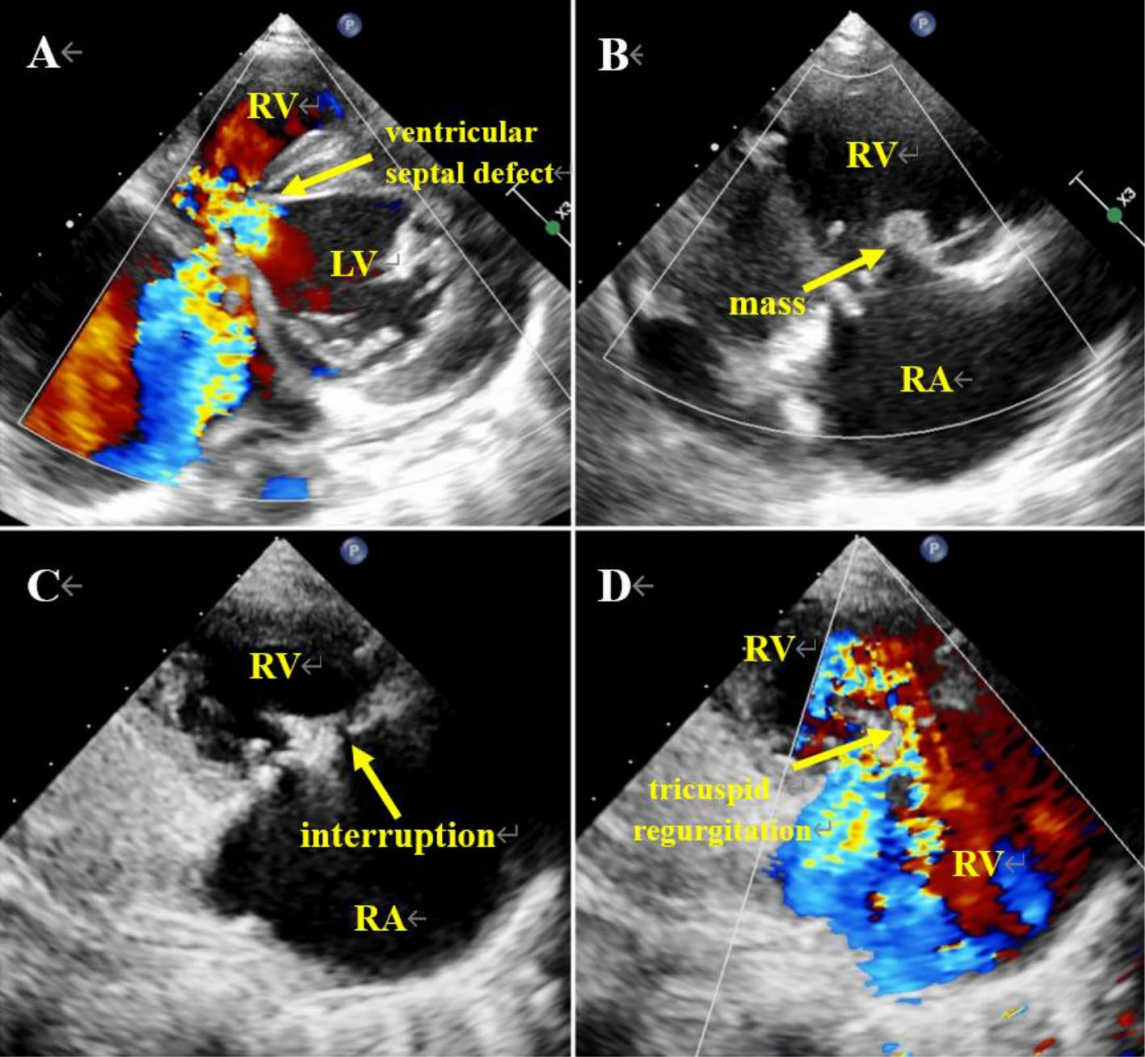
Echocardiography images of Case 1. (A) Color doppler flow imaging (CDFI) shows a ventricular septal defect (VSD) of 0.5 cm (arrow), shunting from left to right. (B) Tricuspid anterior leaflet atrial surface is slightly hyperechoic with a mass of about 0.9 × 0.6 cm (arrow), oscillating with the anterior leaflet. (C) Arrow shows interrupted echo continuity of the anterior lobe of the tricuspid valve (about 0.2 cm). (D) The CDFI shows tricuspid regurgitation, in which a beam of regurgitation comes from the interrupted echo of the anterior lobe of the tricuspid valve (arrow). LV = left ventricle; RV = right ventricle; RA = right atrium
